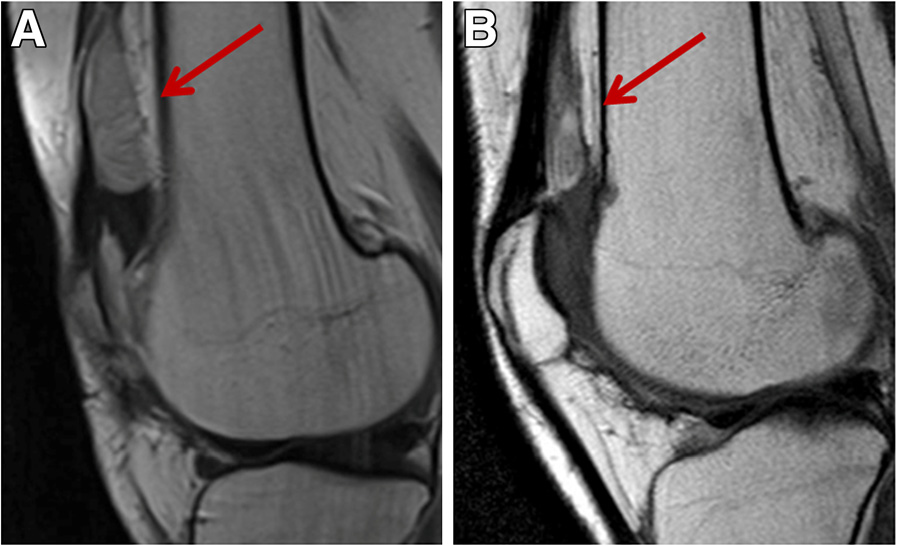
Magnetic resonance imaging (MRI) tumor assessment of Patient 2. Sagittal contrast enhanced (ce) TSE T1 weighted (w) MRI images at baseline showed a lesion located behind the rotula (panel A). After 2 months of imatinib a decrease in tumor size was detected (panel B).
